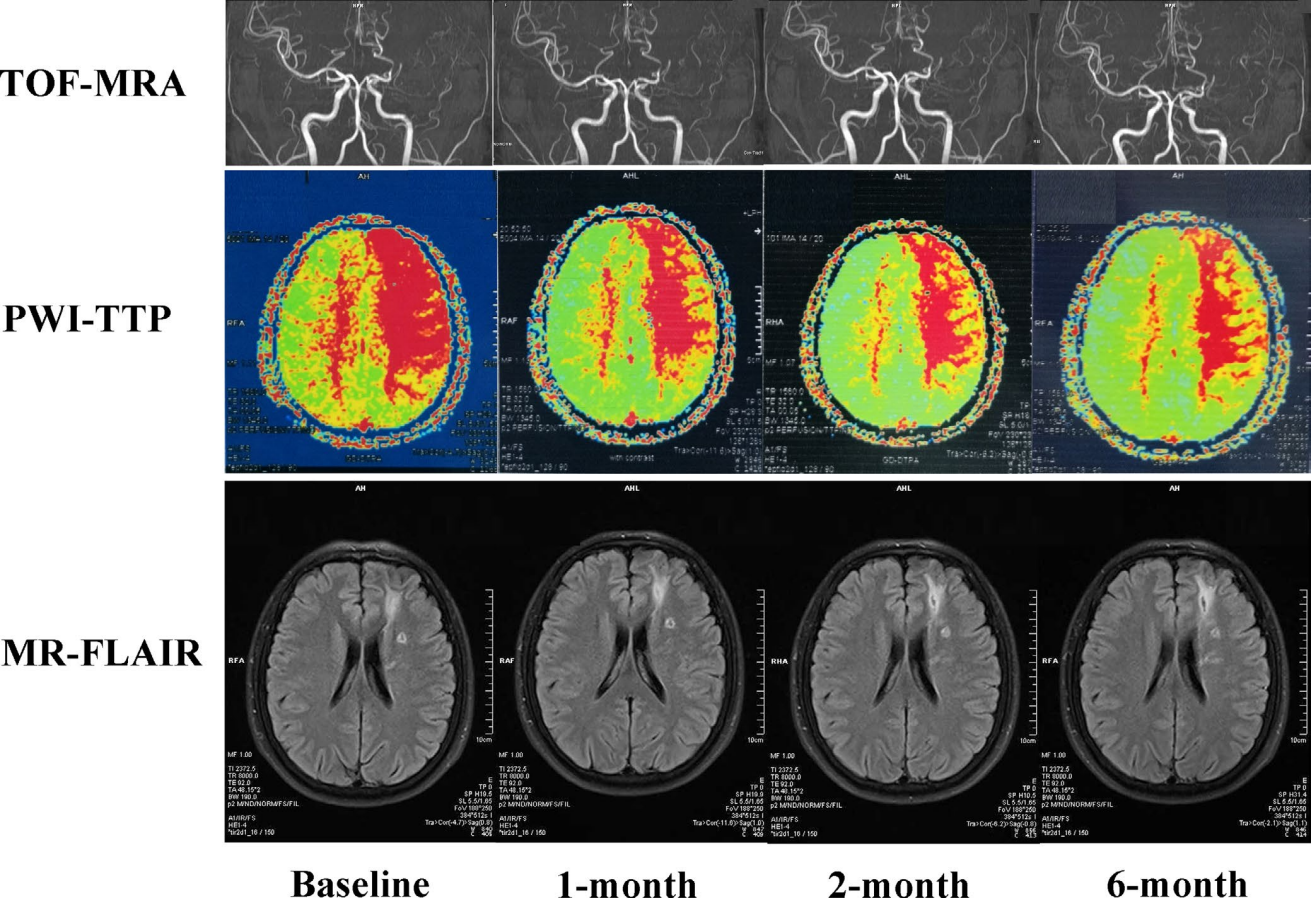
Dynamic changes of MR images of a case at 6 months post‐RIC. A 35‐year‐old male MMD patient with left distal MCA stenosis (TOF MRA) and left frontal infraction (MR‐FLAIR) at baseline prior to RIC and after 1, 2, and 6 months of RIC. PWI showed the degree of prolonged TTP in left frontal and parietal lobe were remarkably attenuated after he underwent 1, 2, and 6 months of RIC, which meant that the collateral circulation formed to shorten the blood transit time TTP values. In the 1, 2, and 6 months of RIC follow‐up time points, there was no recurrent stroke shown on MR‐FLAIR and no progressive stenosis presented on TOF MRA
